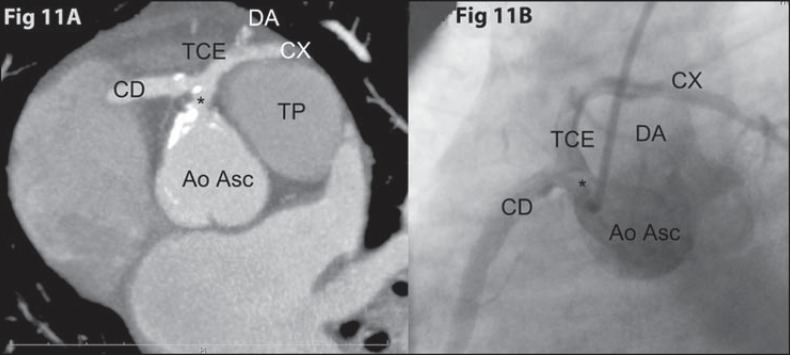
Single coronary trunk*. Fig 11A: Oblique axial coronary computed tomography
